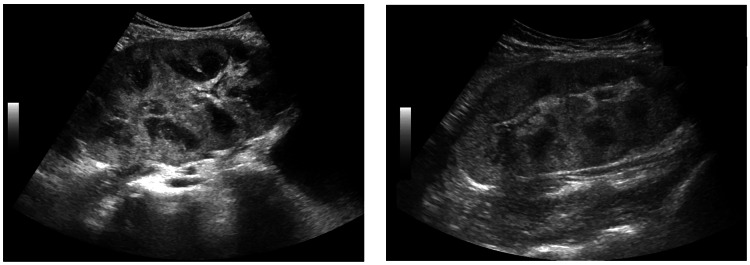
Sagittal views of transplanted kidney on ultrasound showing increased renal echogenicity
